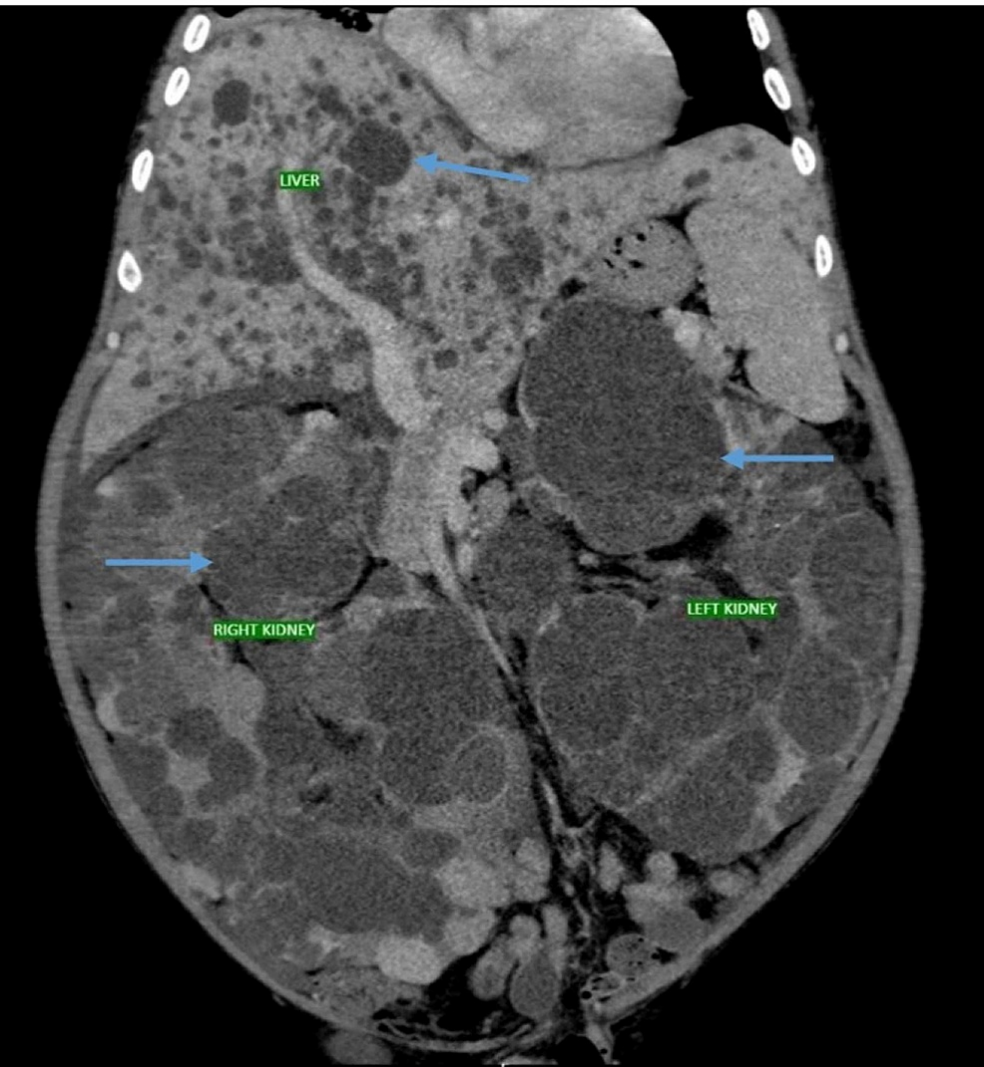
CT abdomen showing multiple varying-sized cysts replacing parenchyma in both kidneys and liver (blue arrows). CT; computed tomography.
